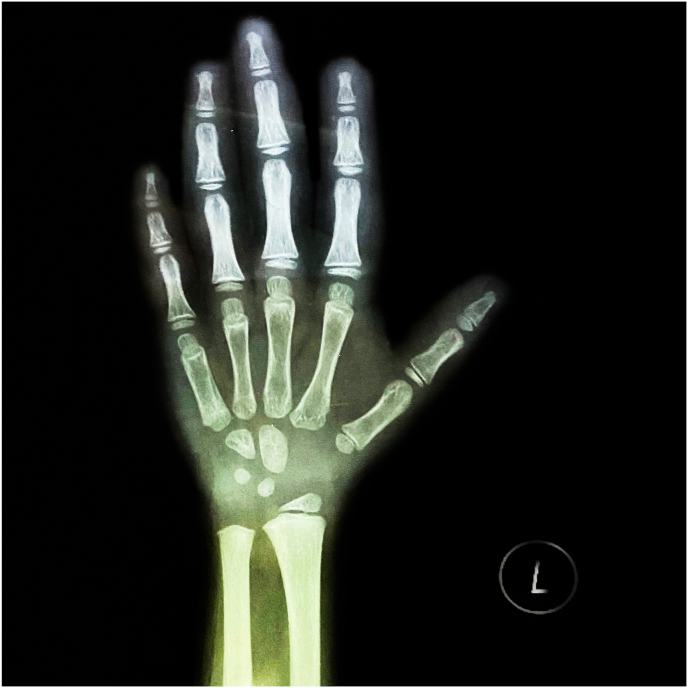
Left hand x-ray showing mild acroosteolysis in the distal phalanges.
